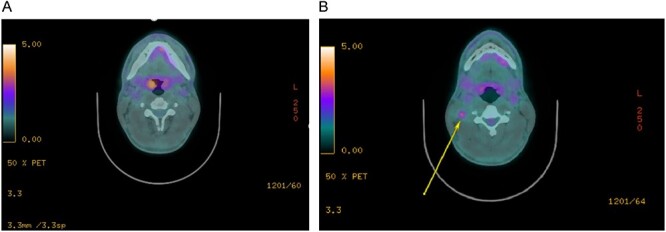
Staging imaging. (A) PET-CT imaging of the right tonsil primary lesion. (B) PET-CT imaging of the right level IIb avid node.
